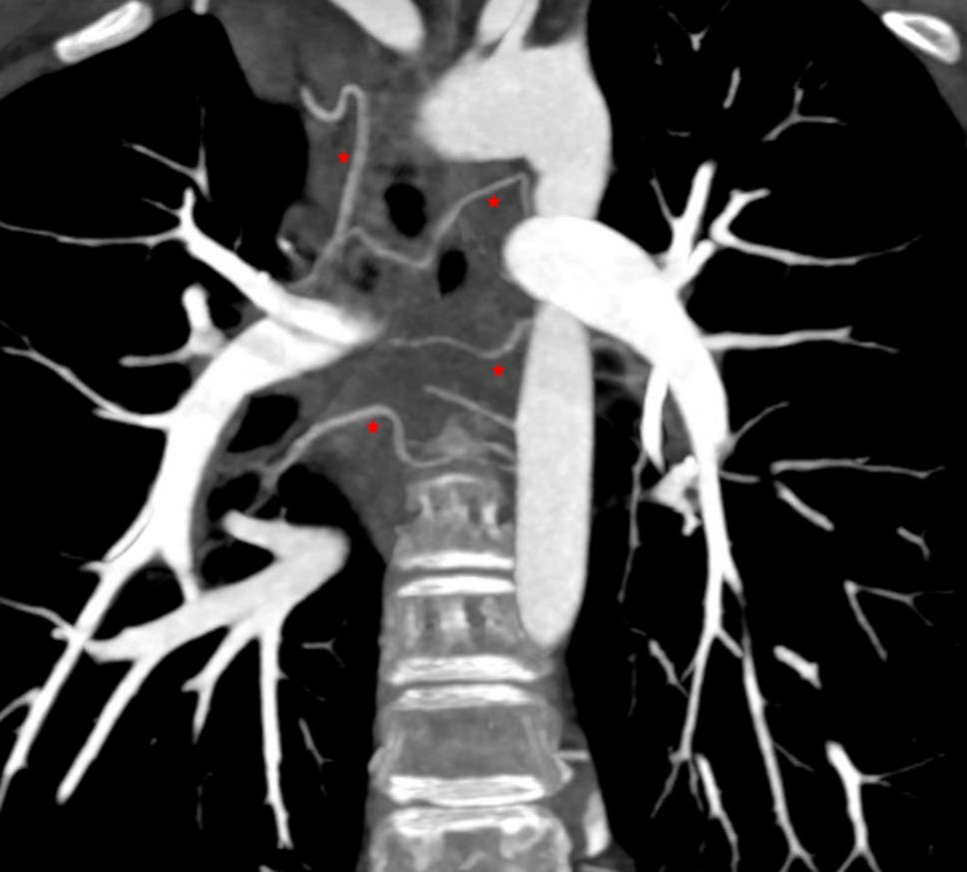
Cardiac CT coronal section, MIP image shows a few major aortopulmonary collaterals (red stars)
